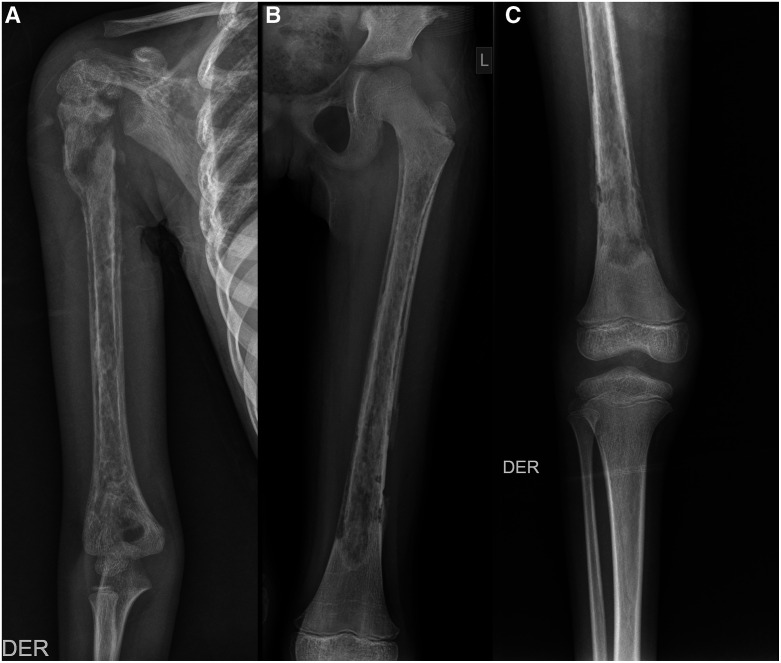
(A) X-ray examination of the right humerus shows osteomyelitis with a pathological fracture of the metaphysis of the humerus and glenohumeral subluxation. (B) X-ray examination of the left femur shows osteomyelitis. (C) X-ray examination of the right femur shows osteomyelitis.
